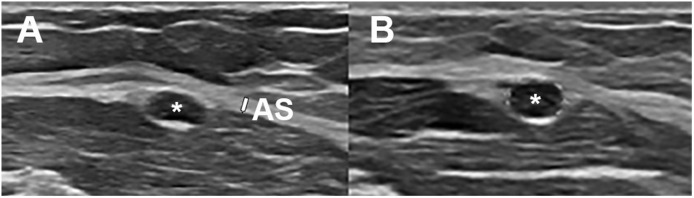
Ultrasound (US) images obtained proximal to the elbow. A: transverse image obtained 5.5 cm proximal to the medial epicondyle. The ulnar nerve (asterisk) passed under a tendinous arcade of Struthers (AS, white arrow) and was oval shaped. B: transverse images obtained just proximal to the arcade of Struthers showed an increase in surface area of the ulnar nerve (asterisk) compared with the level of the arcade.
